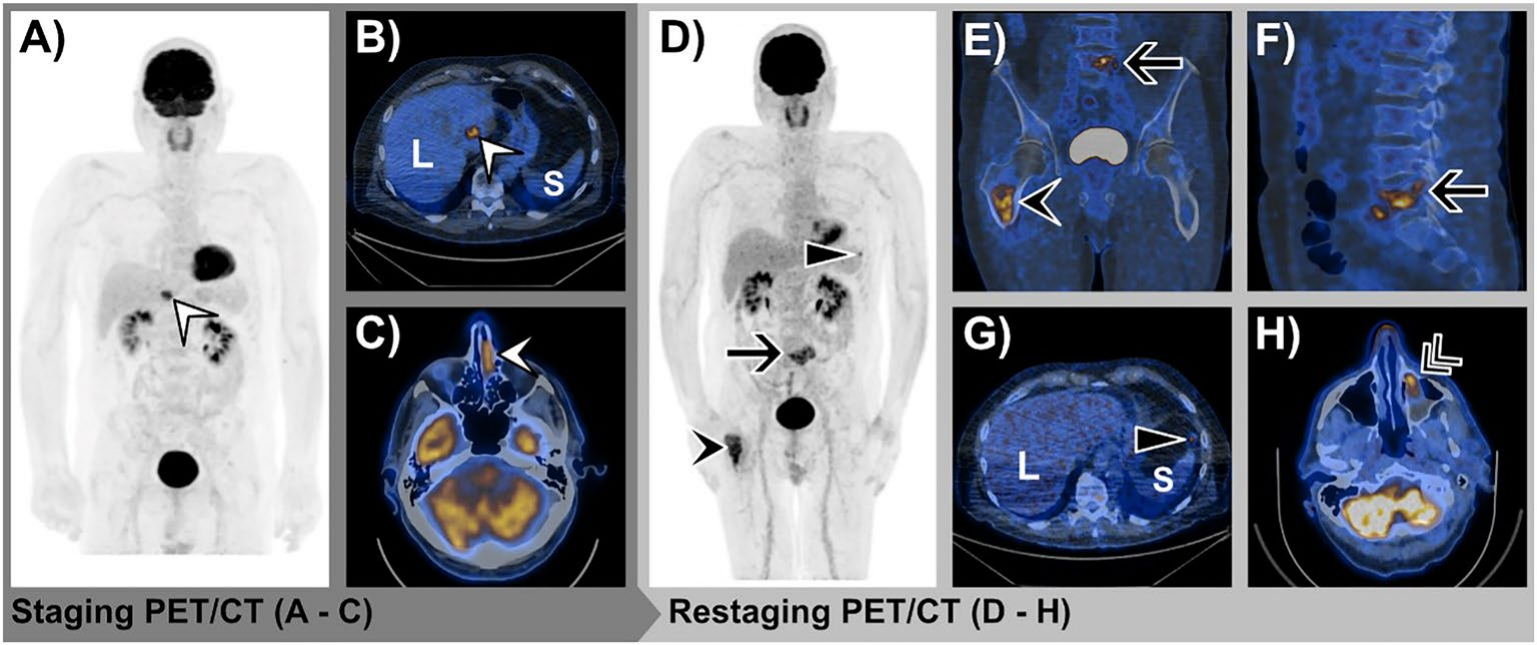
71-year-old female with SMM in the left nasal cavity. Initial FDG-PET/CT staging examination before the initiation of anti-PD-1-based therapy: (A) Whole-body maximum intensity projection (MIP) image shows an FDG-avid metastasis in the left lobe of the liver (white arrowhead). (B) Axial fused FDG-PET/CT confirms the FDG-avid liver metastasis (white arrowhead). (C) Axial fused FDG-PET/CT with the FDG-avid primary in the left nasal cavity (white arrowhead). FDG-PET/CT restaging examination 3 months after the initiation of anti-PD-1-based therapy shows PMD: (D) MIP image shows new FDG-avid soft tissue metastasis anterior to the spleen (black triangle) and a new bone metastasis in the fifth lumbar vertebral body (black arrow) and in the right proximal femur (black arrowhead). (E,F) Axial fused FDG-PET/CT with corresponding bone metastasis in the proximal femur (black arrowhead) and fifth lumbar vertebral body (black arrow). (G) Axial fused FDG-PET/CT with corresponding metastasis anterior to the spleen (black triangle). (H) New FDG-avid metastasis in the left maxillary sinus. FDG-PET/CT; 2-[^18^F]-fluorodeoxy-d-glucose positron emission tomography/computed tomography, *L* liver, *S* spleen, *PMD* progressive metabolic disease, *SMM* sinonasal mucosal melanoma.
